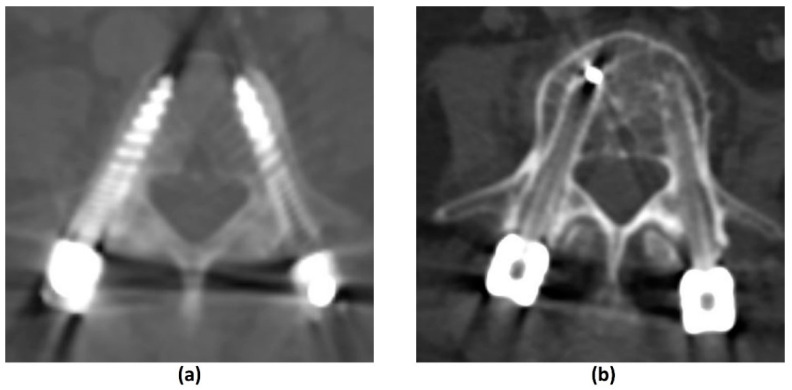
Titanium screw implants (a) and CFR-PEEK implants (b) on CT imaging. CFR-PEEK implants are radiolucent and result in less metal-related artifacts including beam hardening artifacts.
